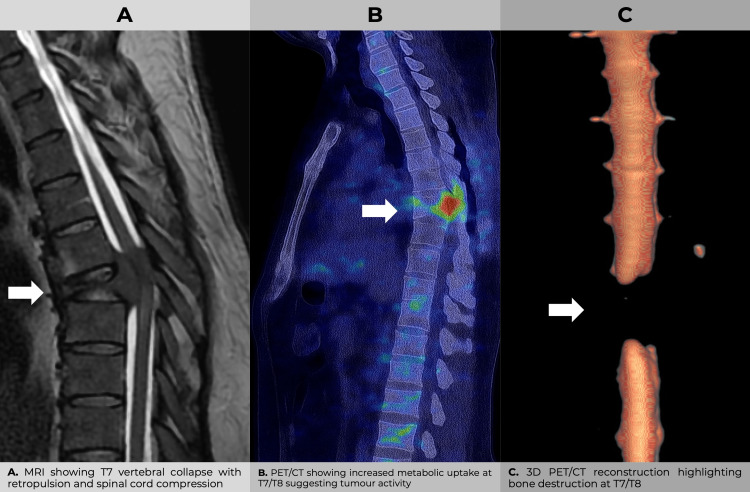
Multimodal imaging of the thoracic spine in a patient with T7 vertebral involvement. Sagittal MRI, PET/CT and 3D reconstructions show structural collapse, metabolic activity and bone destruction at the T7/T8 level. These findings collectively support tumour-related vertebral damage with spinal cord compromise.
